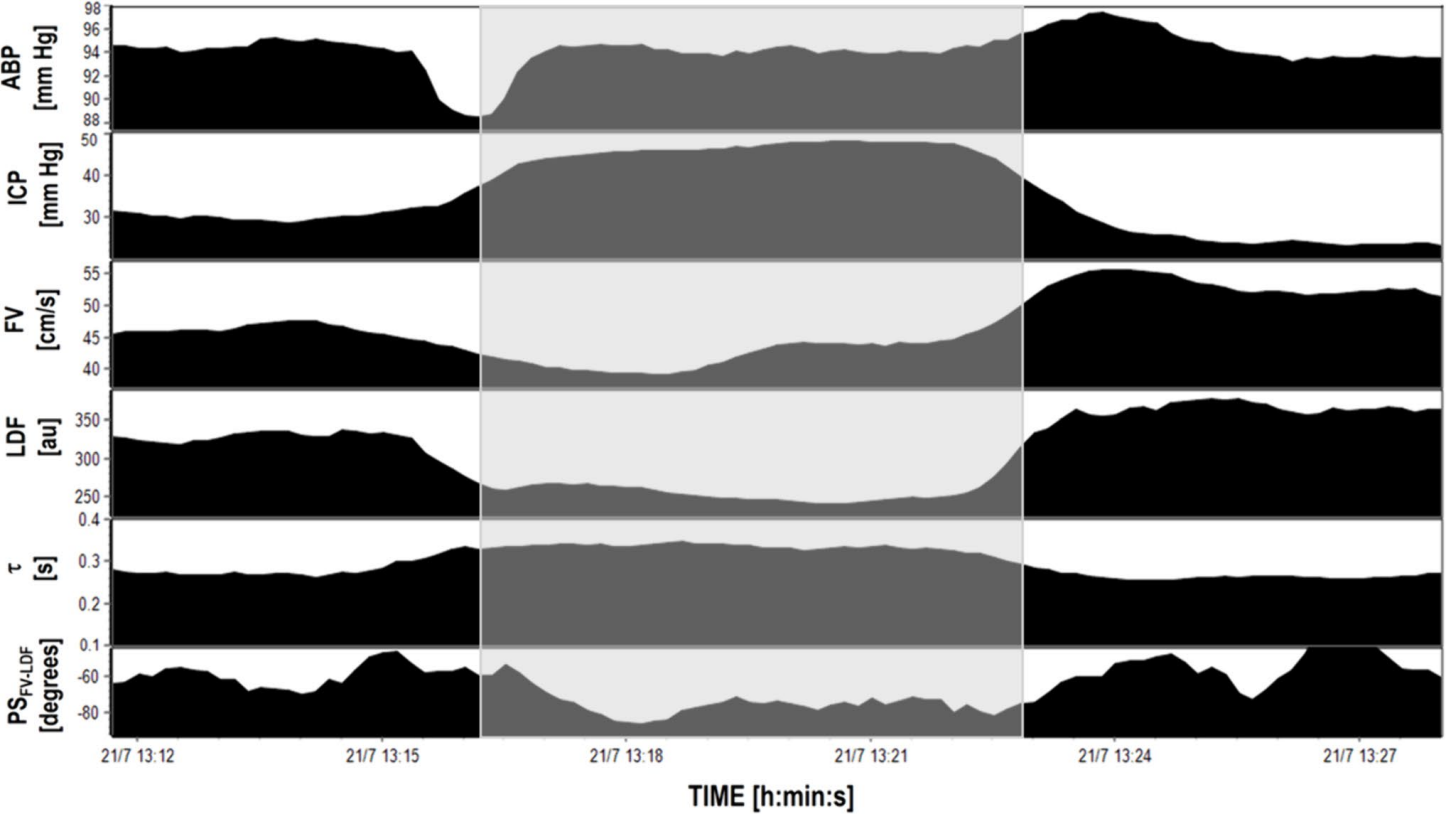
Changes in ABP, ICP, FV, LDF, τ, and PS_FV-LDF_ during an ICP plateau wave recorded in a single TBI patient. With rising ICP during the plateau wave (*area shaded in grey*) PS_FV-LDF_ became more negative while τ increased indicating a greater separation between FV and LDF pulses and a slower transition of blood volume throughout the cerebrovascular bed from macro to microvasculature, during one heart cycle, respectively. Abbreviations: ABP – arterial blood pressure [mm Hg], ICP – intracranial pressure [mm Hg], FV – cerebral blood flow velocity [cm/s]; LDF – laser Doppler flux signal [arbitrary units, au], τ – cerebral arterial time constant [s], PS_FV-LDF_ – phase shift between FV and LDF pulse waveforms [degrees]
